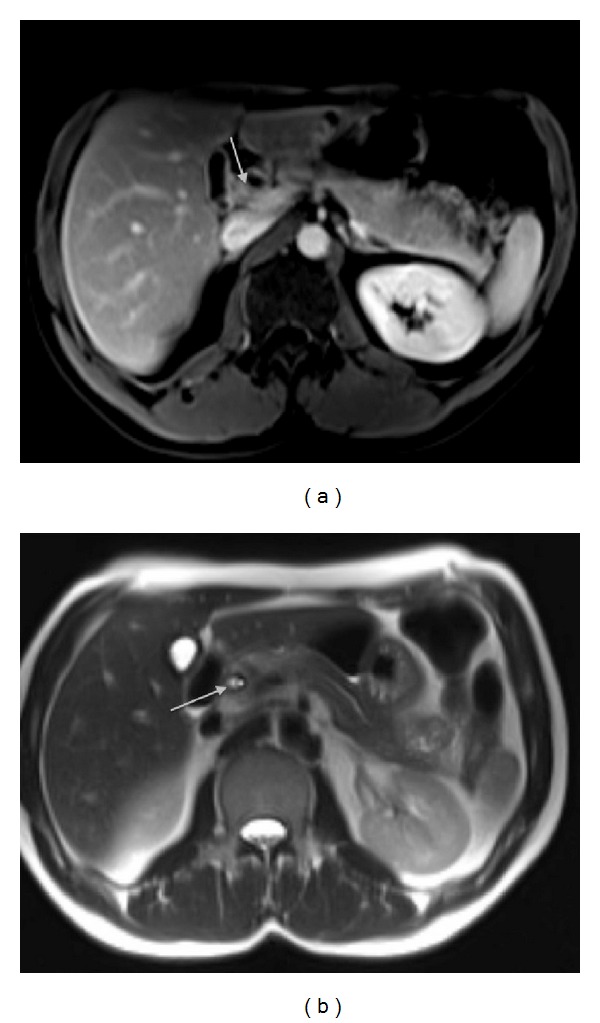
(a) T1 weighted axial postcontrast MRI, asymmetric contrast-enhancing lesion in distal choledoch, and indentation to portal vein (white arrow) ans (b) T2 weighted axial MRI, mild wall thickness in distal choledoch (white arrow).
